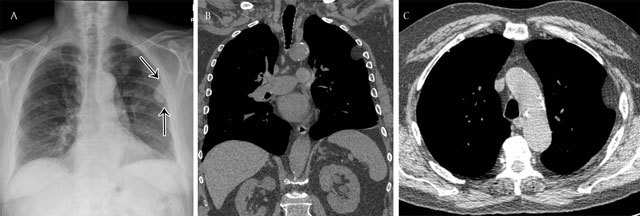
Pleural lipoma in a 79-year old male – Frontal chest radiograph (a) shows a homogeneous opacity with smooth margins, a sharply defined medial edge, and an ill-defined lateral border (black arrows). A coronal reformat CT view in mediastinal window (b) clearly shows the pleural based lesion (b). The axial CT view (c) shows the smooth marginated lesion forming obtuse angles with the chest wall. Note the fat attenuation of the pleural-based lesion.
